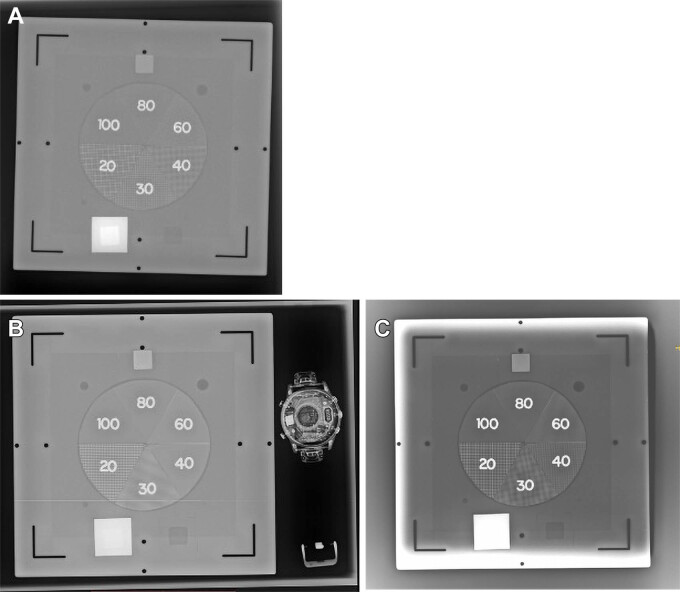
Preflight, in-flight, and postflight nonmedical radiographs. Radiographs
